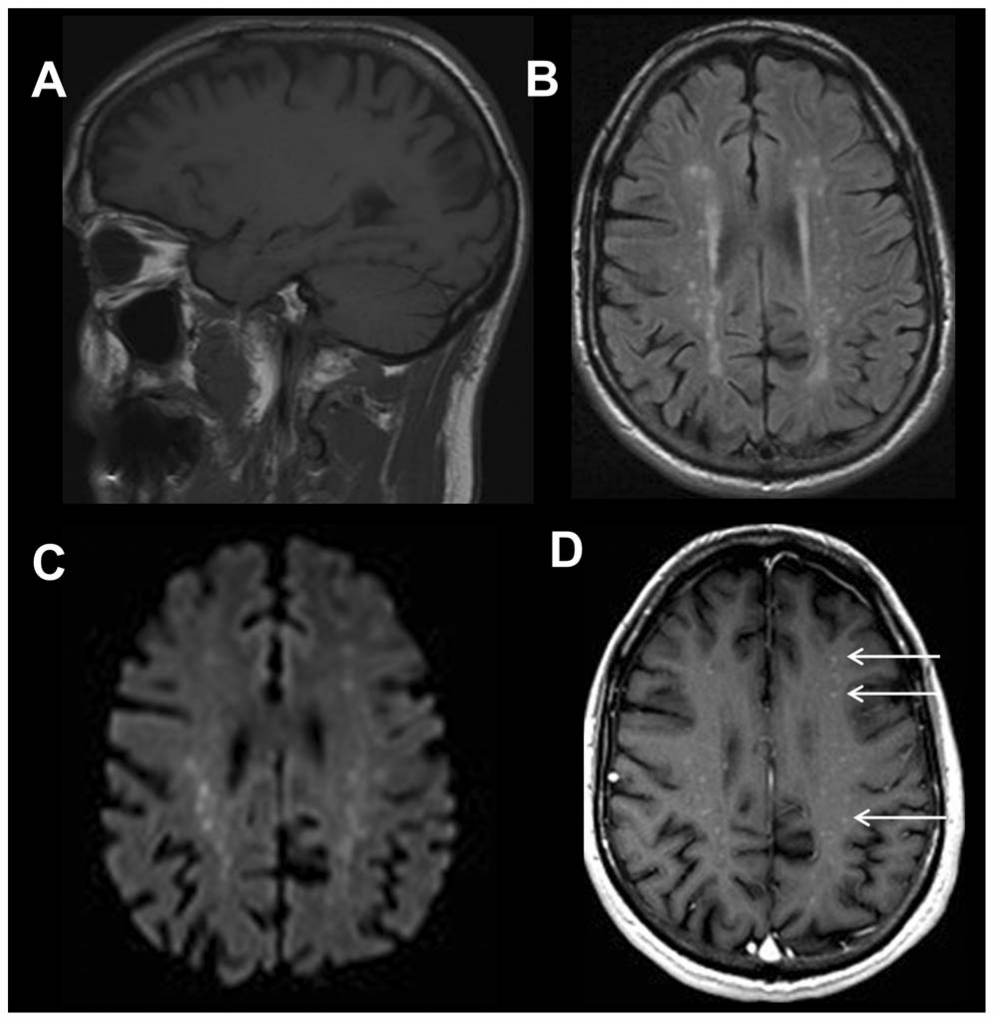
Foci of enhancement in a 57 year old male with ataxia. Sagittal T1-weighted image (A) shows no definite abnormality. Axial T2-weighted FLAIR image (B) demonstrates multiple punctate hyperintense foci involving the deep and periventricular white matter of the bilateral cerebral hemispheres with corresponding restricted diffusion on the axial diffusion weighted trace (C) image. Axial T1-weighted post contrast image (D) reveals numerous punctate foci of enhancement corresponding to the deep and periventricular white matter lesions (white arrows). In this patient, the differential diagnosis included intravascular lymphoma, granulomatous disease, neurosarcoidosis, and vasculitis.
